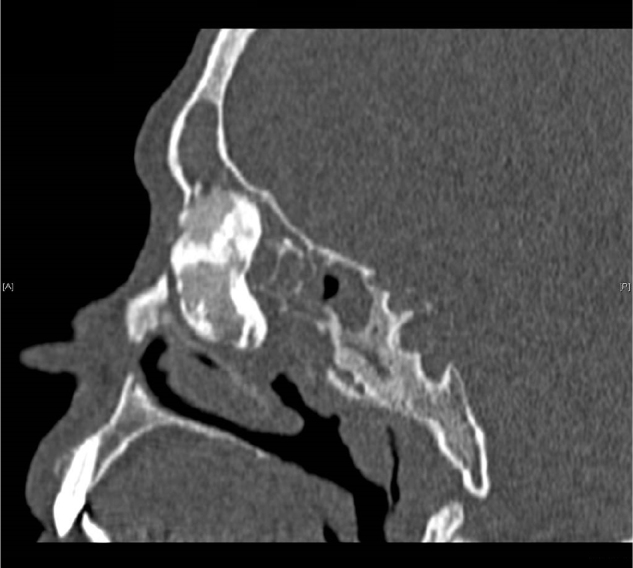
CT scan of the sinuses – giant fronto-ethmoidal osteoma, sagittal plane.
